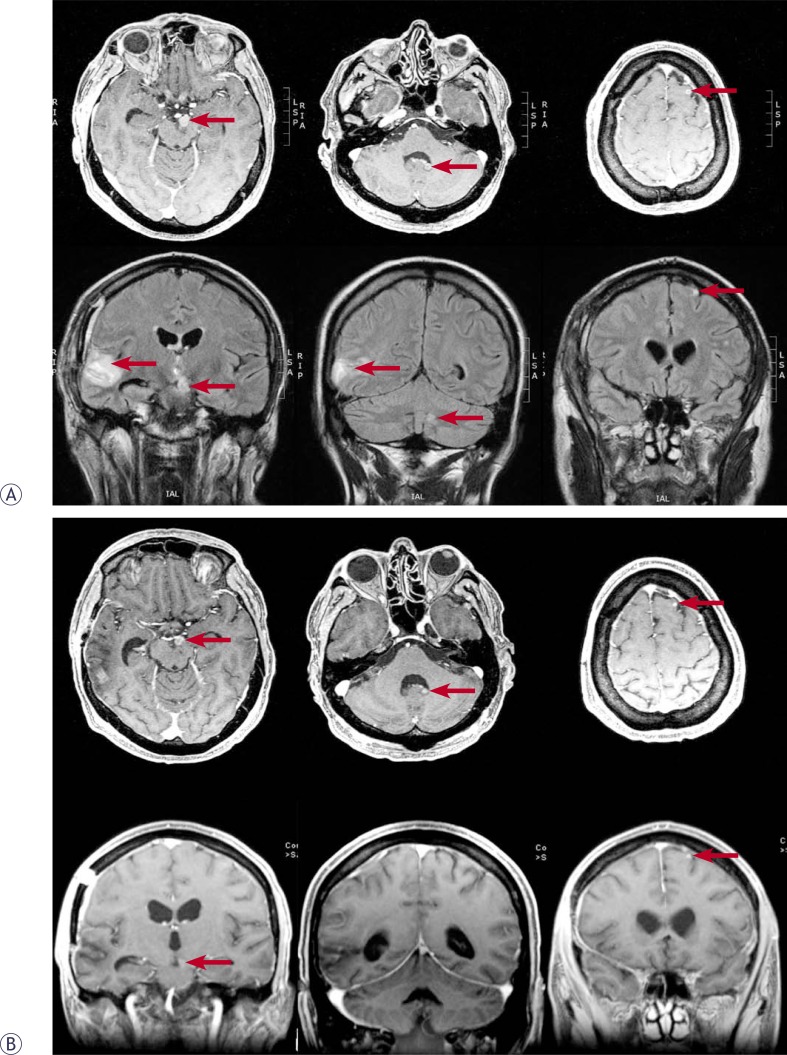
Stereotactic radiosurgery. This radiotherapy technique is characterized with maximal accuracy and is used for focal irradiation of small brain lesions (usually up to 3 tumors of 3.5 cm maximal diameter). After rigid fixation of the head with specific frame, several small beams coming from various directions are focused on one spot inside of the target, creating a steep dose gradient on periphery of the target. Tumor doses in the range of 16–25 Gy are prescribed on 80% isodose encompassing the lesion, whereas 1–2 mm from the edge of the target, the dose drops to 20–30% of its prescribed value. Local control is in the range of 90% and the prevailing cause of death is progression of extracranial disease. In January 2009, a 59-year-old male with melanoma, diagnosed 4 years earlier, presented with 4 metastatic lesions in the brain. On PET-CT, two additional metastases were identified elsewhere in the body, occupying the third lumbar vertebra and the musculature of the posterior abdominal wall. T1-weighted post-contrast MRIs revealed a lesion of 30x20 mm (long arrow) in the right temporoparietal region, a smaller one in the left half of the pons (short arrow), a 6 mm lesion in the left frontal lobe (thick arrow) and a 7 mm lesion in the left cerebellar hemisphere (arrowhead) (Figure 2A). The patient was treated with surgical resection of the large temporoparietal metastasis, whole brain irradiation (10 × 3 Gy), temozolamide and stereotactic radiosurgery of other three (smaller) brain metastases with the irradiation doses to 80% isodose of 20 Gy (the lesion in the frontal lobe) and 18 Gy (the lesions in the pons and cerebellum). Four months after the procedure, the size of all three irradiated tumors was reduced and no new lesion was identified in the brain (Figure 2B). In September 2009, disease progression was recorded after detecting a metastasis in the spinal cord which was treated with surgery, postoperative irradiation and chemotherapy. No progression of treated brain metastases occurred so far (January 2010, 11 months after stereotactic radiosurgery).
